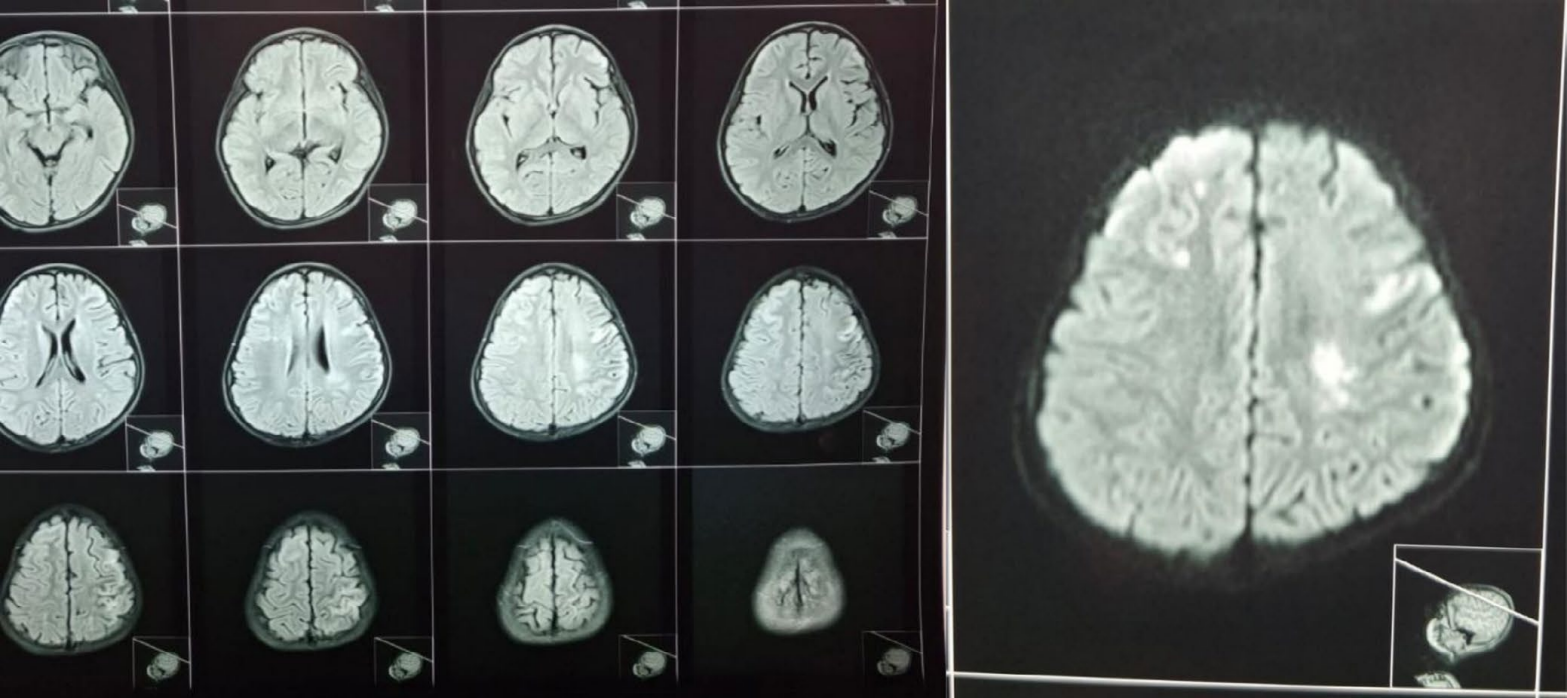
MRI multiple acute infarcts in the bilateral frontal and left parietal lobes.
